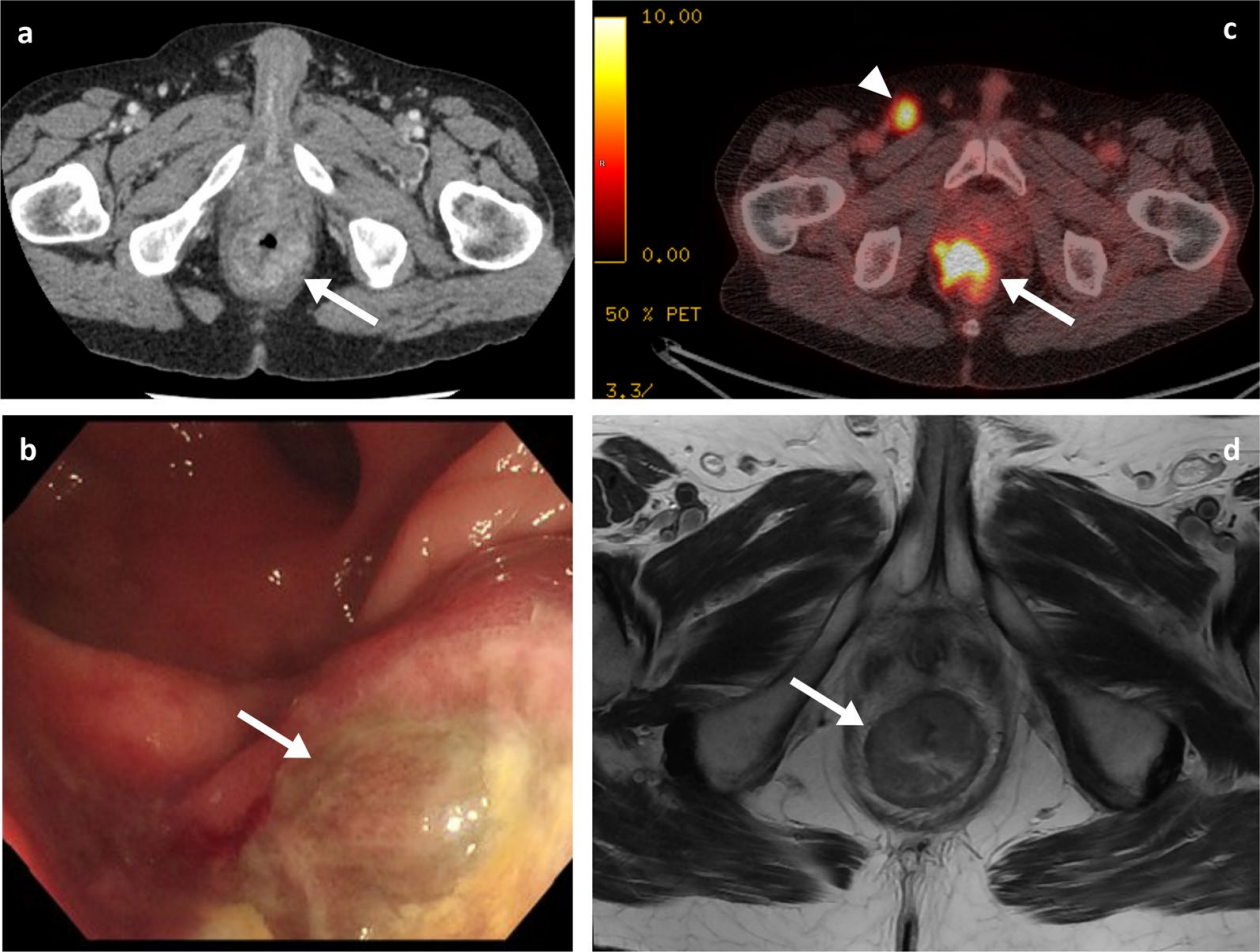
Portal phase contrast-enhanced CT axial (a), colonoscopy (b), PET-CT axial (c) and MRI T2WI axial (d) of a 64-year-old male patient with an infiltrative metastatic anorectal melanoma mass. The PET-CT (c) also demonstrates a pathological right inguinal node (arrowhead)
